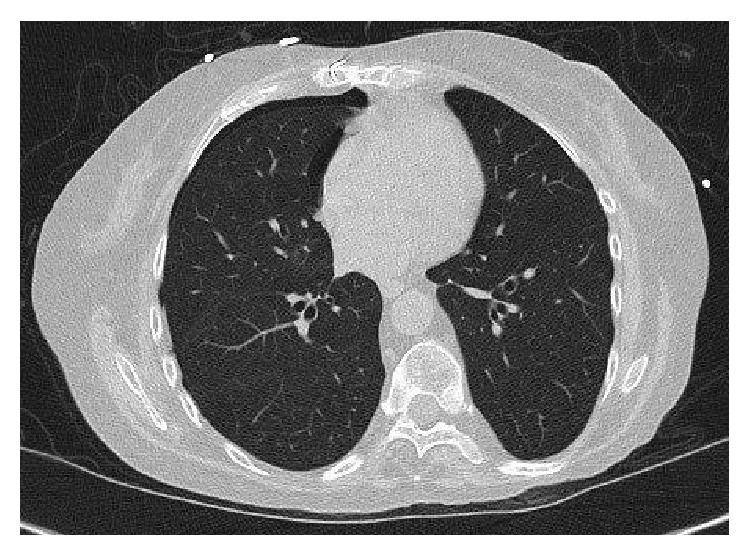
Computed tomography scan of the chest revealing resolution of nodule at 3 months.
